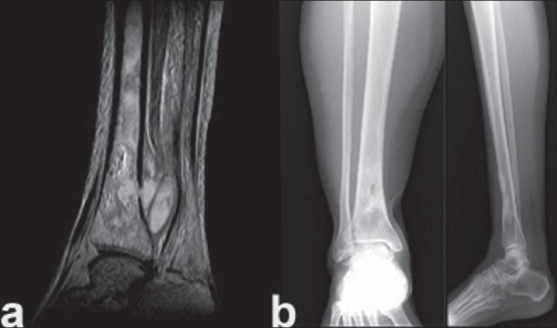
T2W MR image of tibia showing (a) osteomyelitis of tibia with abscess in soft tissue (b) X-ray anterposterior and lateral view of tibia showing healed osteomyelitis of lower end tibia after 28 months
